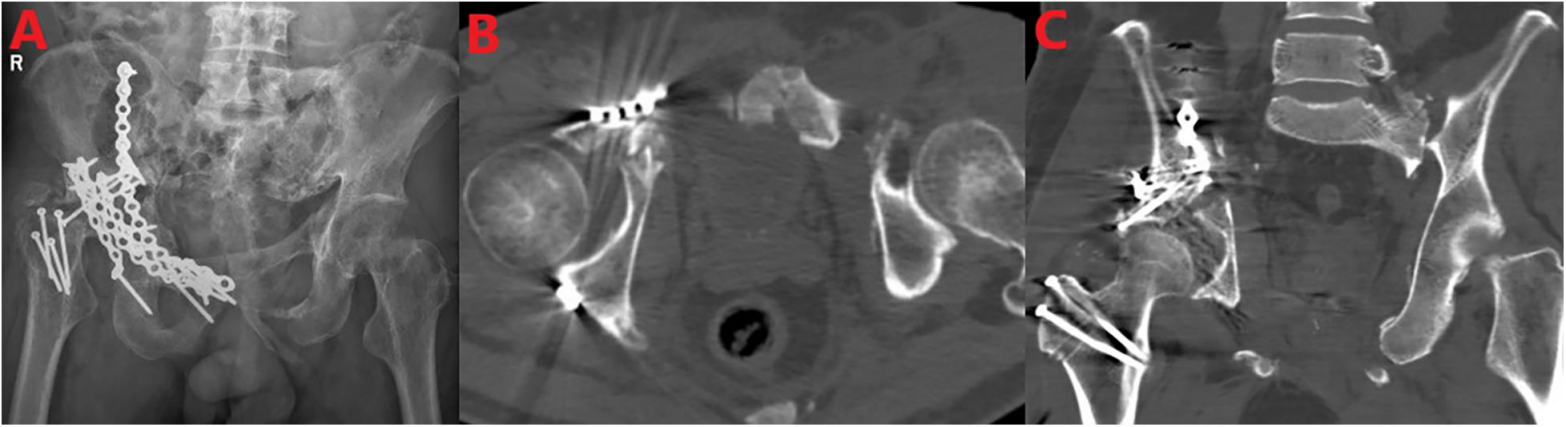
X-ray (A) and CT [(B) axial view, (C) coronal view] showed postoperative internal fixation of multiple pelvic and femoral fractures, with no obvious dislocation or fracture of the internal fixation. The pictures highlight the correct position and effectiveness of the internal system components at the surgical site.
